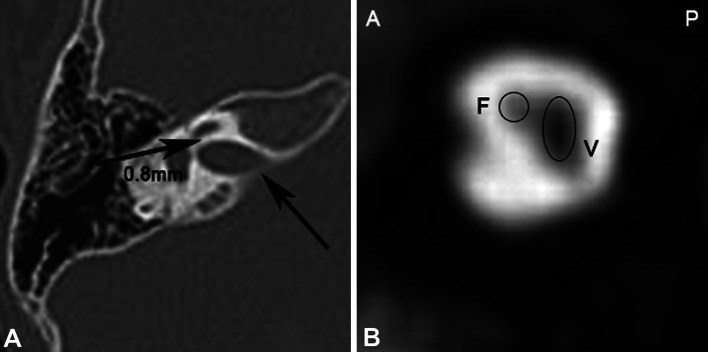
Normal IAM with an aplastic CN. Female, 2 years old. The right ear has total hearing loss and the left ear has normal hearing. a Axial CT shows a normal IAM. The diameter of the CNC is 0.8 mm (*arrow*). b Oblique sagittal reconstruction of an MRI shows the FN and vestibular nerve in the IAM. The CN is absent
